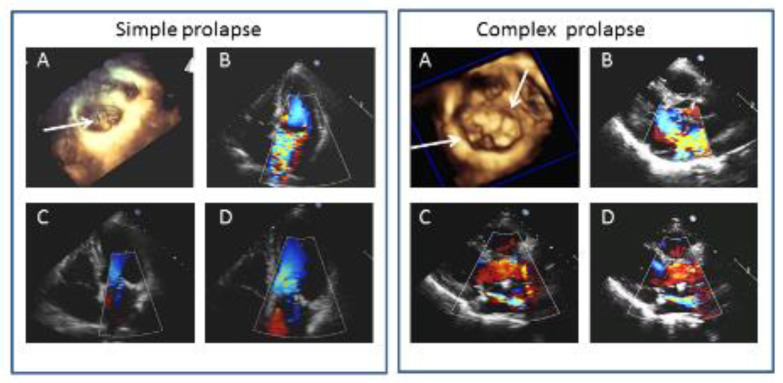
Left panels: Example of simple mitral valve prolapse (MVP). (A) surgical view of three-dimensional MVP reconstruction showing P2 prolapse with multiple chordal ruptures (arrow). (B) two-dimensional transthoracic 4 chamber apical view showing a severe mitral regurgitation (MR). (C,D) two-dimensional transthoracic 4 chamber apical views at 6M and 3Y follow-up, respectively, with trivial MR. Right panels: example of complex MVP. (A) surgical view of three-dimensional MVP reconstruction showing A2, A3, P2 prolapse (arrows) (B) two-dimensional transthoracic parasternal view showing severe MR central jet. (C,D) two-dimensional parasternal long axis views at 6M and 3Y follow-up, respectively, with mild to moderate MR.
